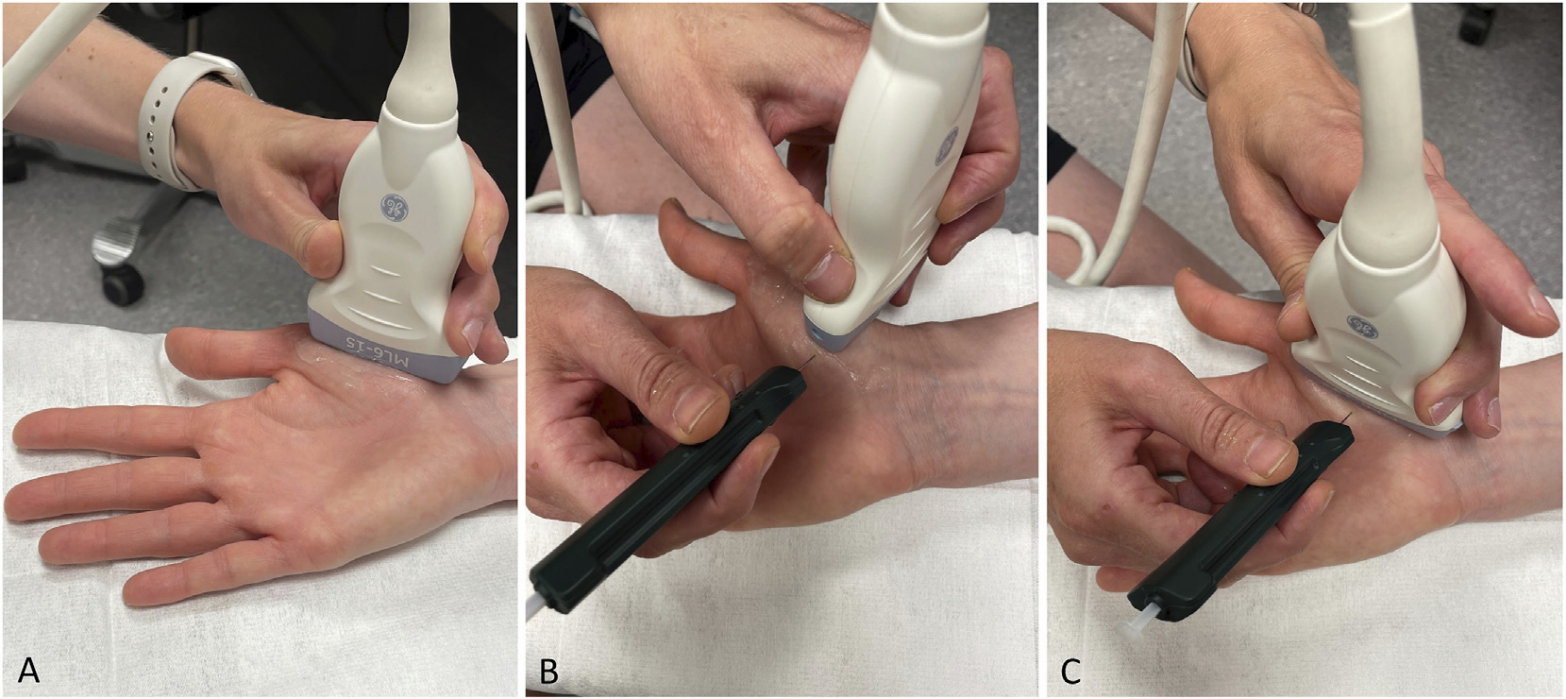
Intraarticular ultrasound-guided injection in the CMC-1 joint.
